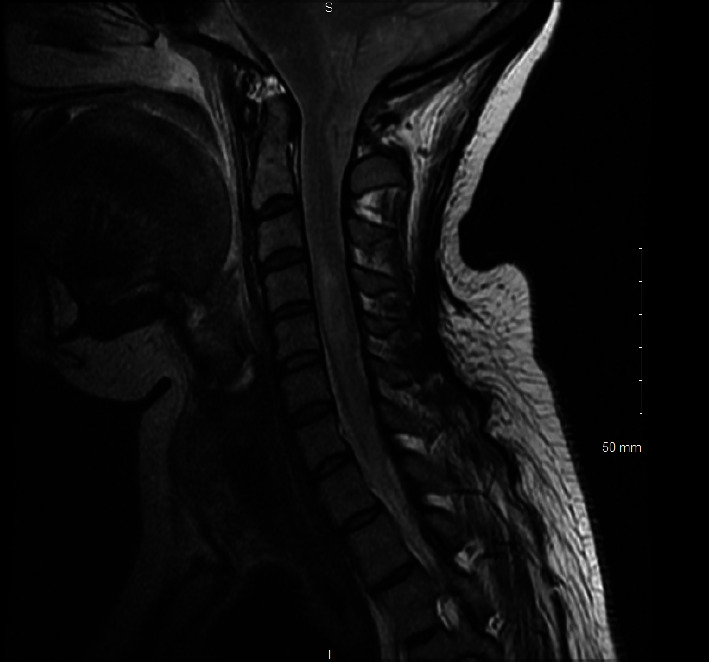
MRI C-spine, sagittal T2 sequence.
